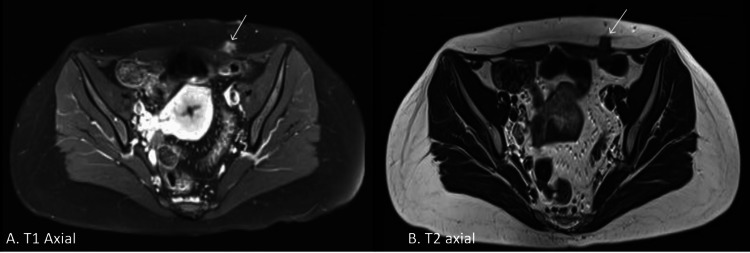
MRI of the soft tissue of the abdominal wall showing a soft tissue lesion in T1 axial (A) and T2 axial (B) sections.
